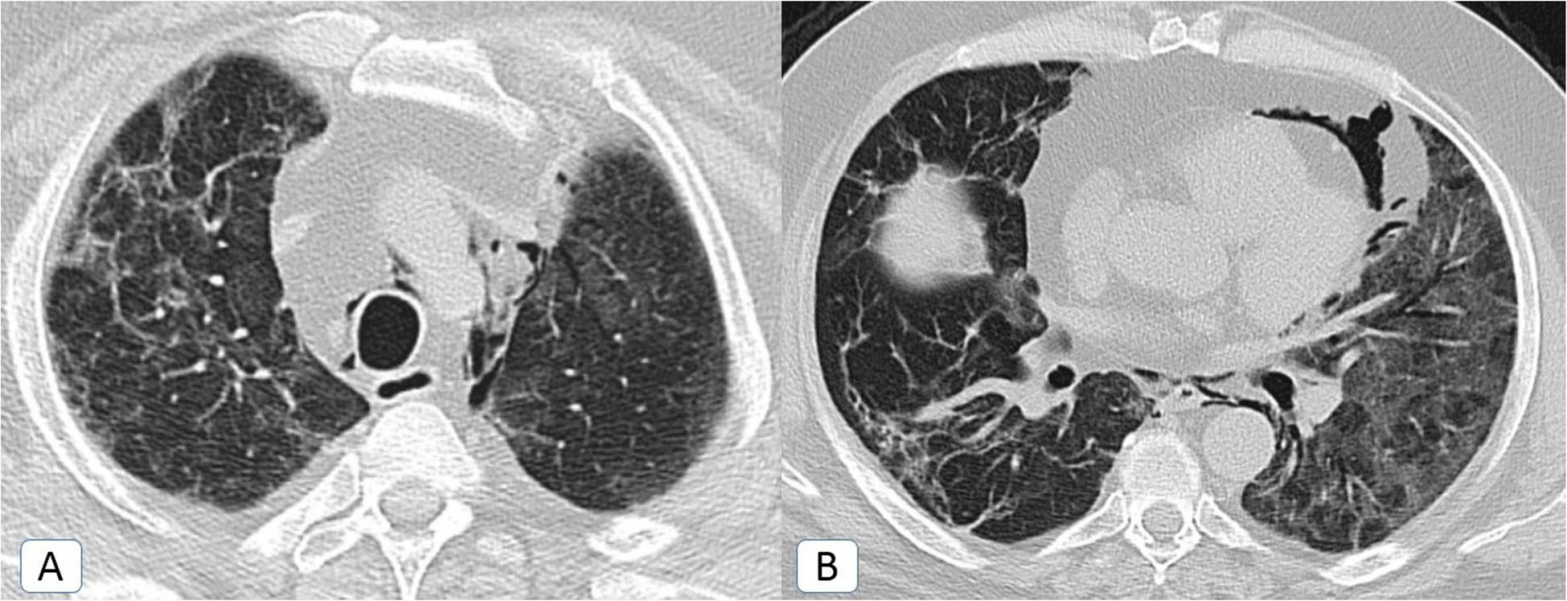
COVID-19 infection complicated by spontaneous pneumo-mediastinum: a 44-year-old male COVID-19 patient. A, B Axial chest CT (lung window) revealed spontaneous pneumo-mediastinum with bilateral ground-glass patches and fibro-atelectatic bands of COVID-19 infection. Minimal associated pneumo-thorax was also there
